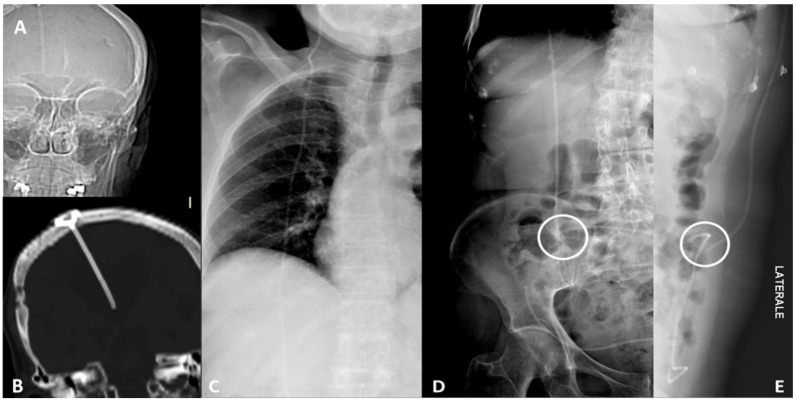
Device integrity. Ventriculoperioteneal shunt malfunction in a patient with history of hydrocephalus. CT scout view (A) and coronal CT scan (B) of the head show the correct placement of the tip of the shunt inside the right lateral ventricle. Frontal chest radiograph (C) shows the integrity of the device along its thoracic course. Frontal (D) and lateral (E) abdominal radiographs detect a fracture of the device in the right lower quadrant of the abdomen.
